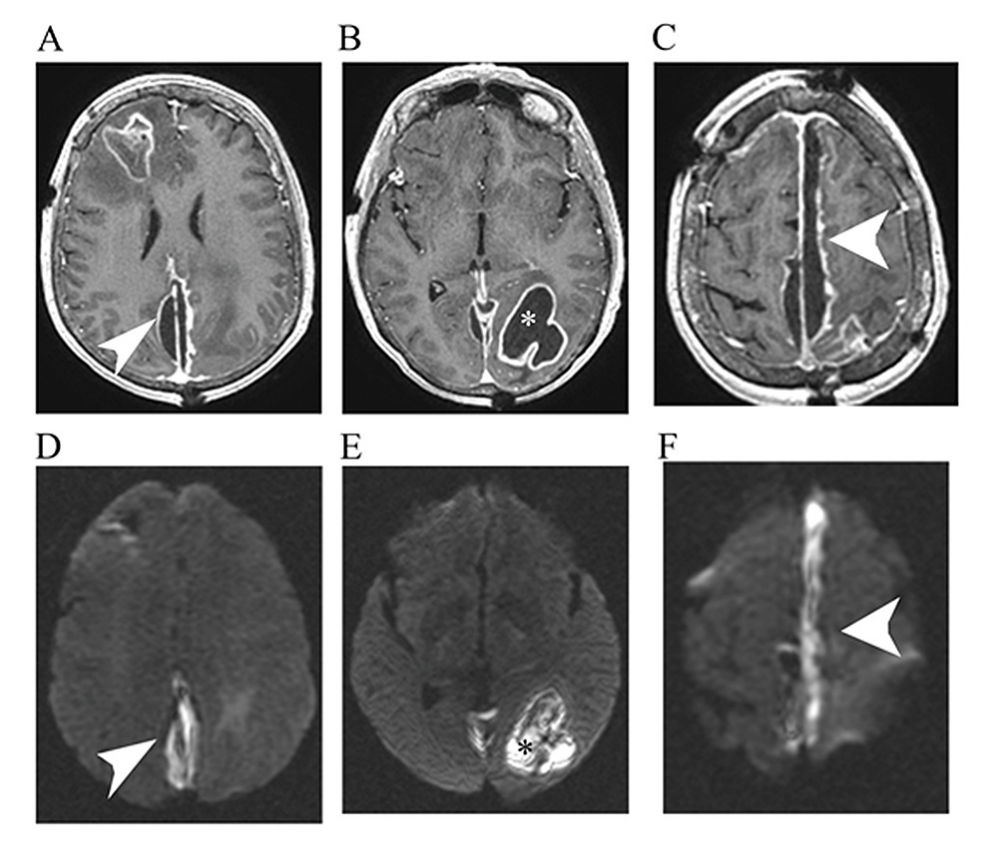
Repeat MRI with contrast T1. MRI of the brain axial with contrast T1 sequence (A-C) and DWI sequence (D-F) with new subdural empyema (arrows) and worsening of the occipital abscess are shown, suspected of communications between these lesions. MRI: magnetic resonance imaging; DWI: diffusion-weighted imaging
